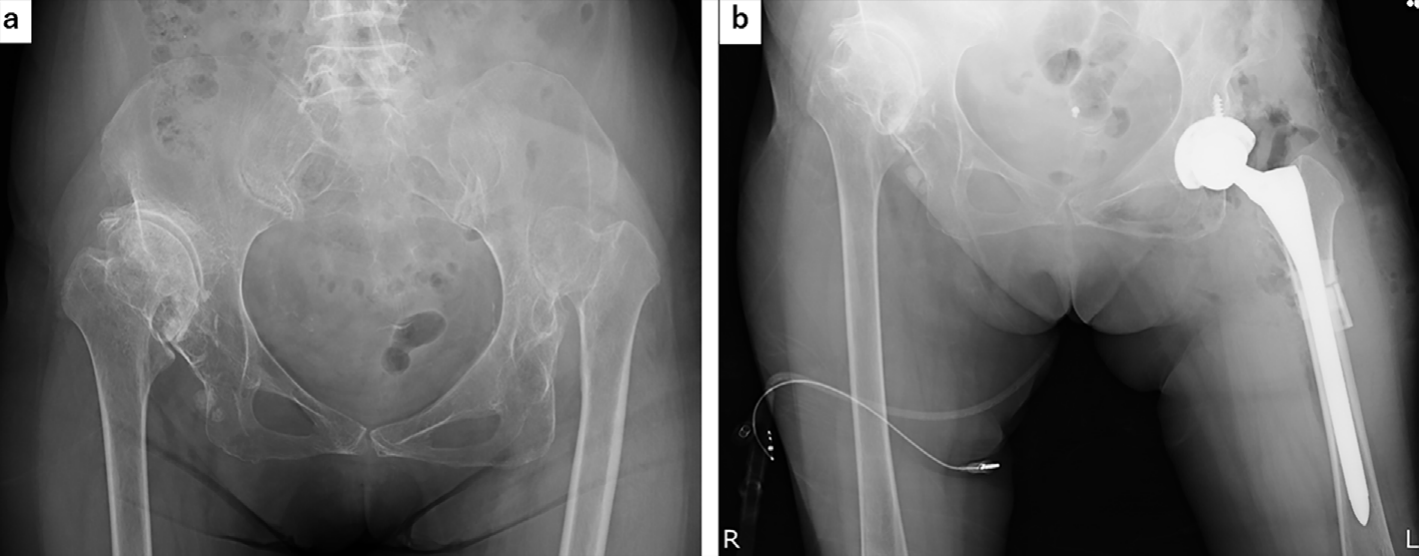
(a) Preoperative X-ray image. Bilateral developmental dysplasia of the hip. (b) Postoperative X-ray image. If the shortened femoral bone fragment is fixed to the osteotomy with a cable, it can sufficiently withstand full weight-bearing walking immediate weight bearing.
